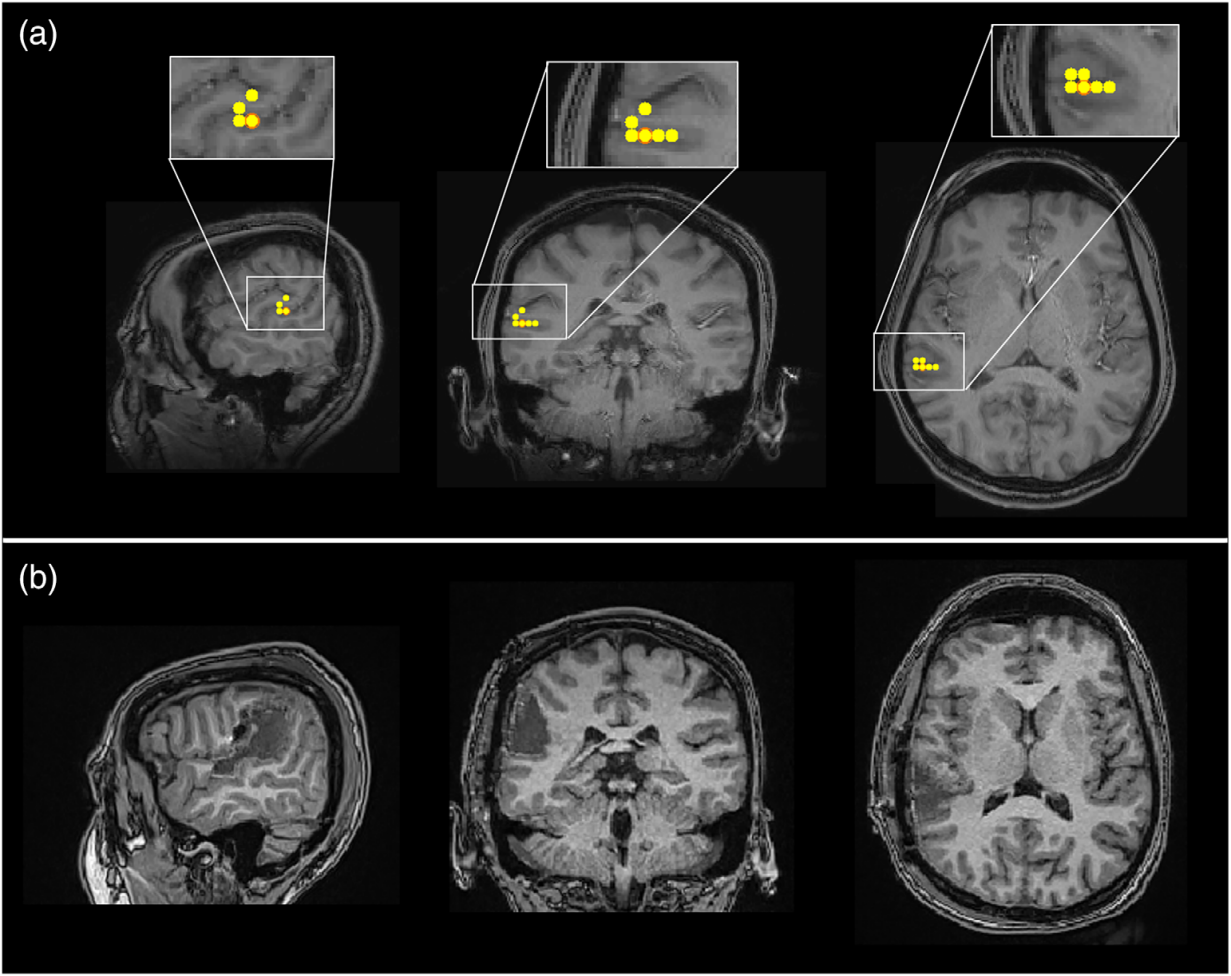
Epilepsy Case 2—magnetoencephalography (MEG) localizations compared with resected area for Patient 5 (focal polymorphic). Panel (a) shows the MEG localizations (peak virtual electrode locations) for the excess kurtosis mapping method (red markers) and the hidden Markov model method (yellow markers). Note that markers may be overlaid so that red markers are hidden under yellow ones. Panel (b) shows the resected area in the postoperative magnetic resonance imaging
